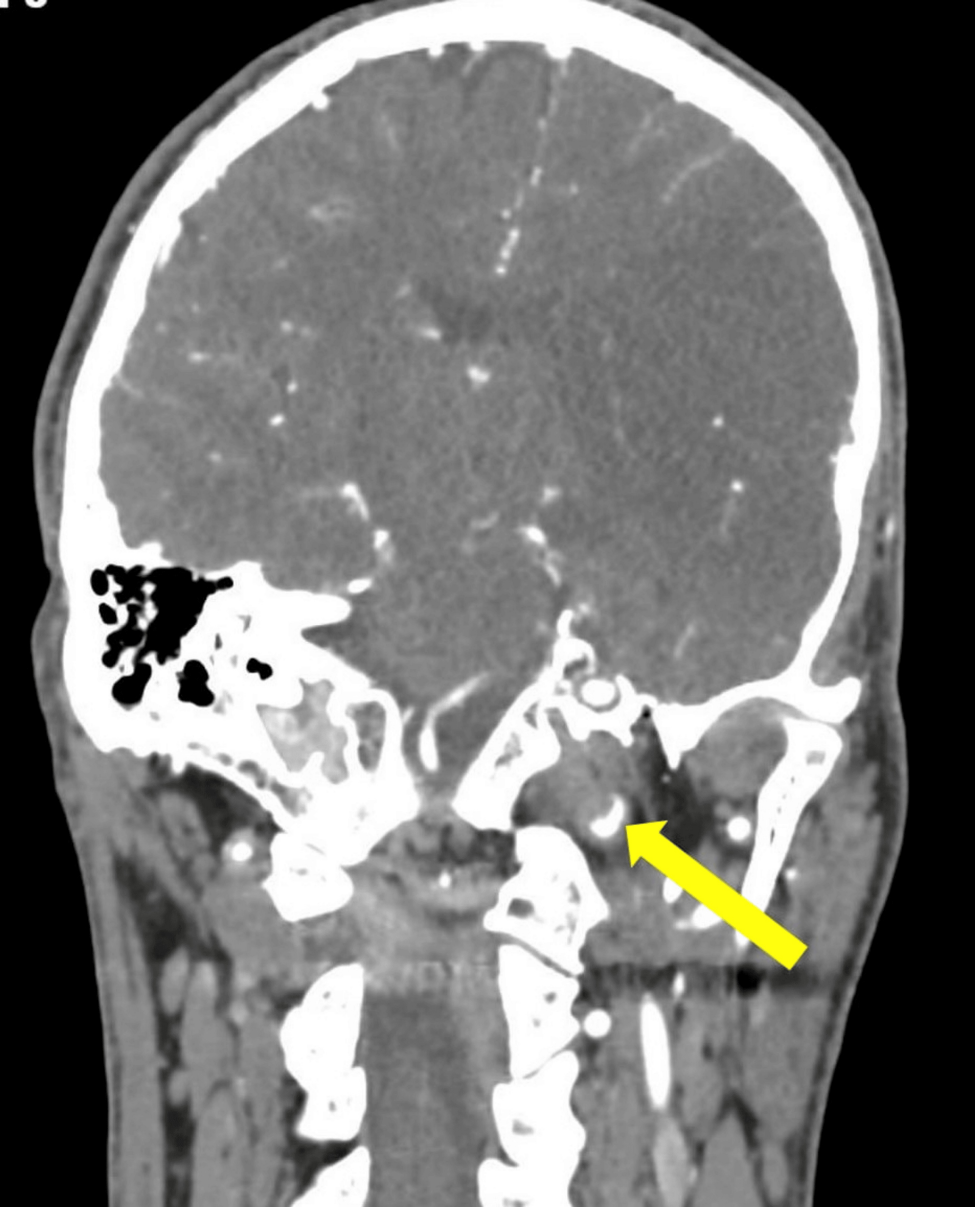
Coronal CT angiogram showing dissection of the cervical part of the left ICA CT: computed tomography, ICA: internal carotid artery
